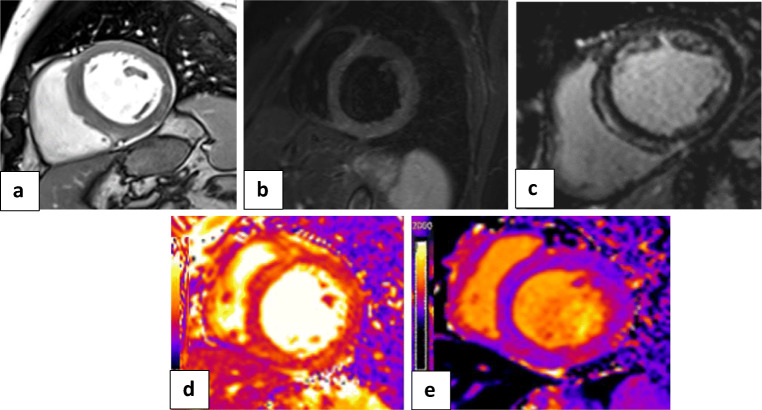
Cardiac MRI: a white blood image 2-chamber short-axis view showing preserved shape and configuration of the left ventricle. b T2-weighted-STIR image 2-chamber short axis showing faint patchy interstitial edema of the myocardium. c IR post-contrast image 2-chamber view showing multiple patchy mid-myocardial enhancing zones of the late gadolinium enhancement, more evident at basal septal and inferior walls. d, e T2 and T1 cardiac MRI mapping sequence in two-chamber view respectively showing intense interstitial myocardial edema with native T2 = 67 ms and elevated T1 value = 1154 ms. Conventional coronary angiography of LAD confirmed normal appearance with no stenosis or occlusion. The final diagnosis was acute myocarditis COVID-like syndrome (AMCovS)
